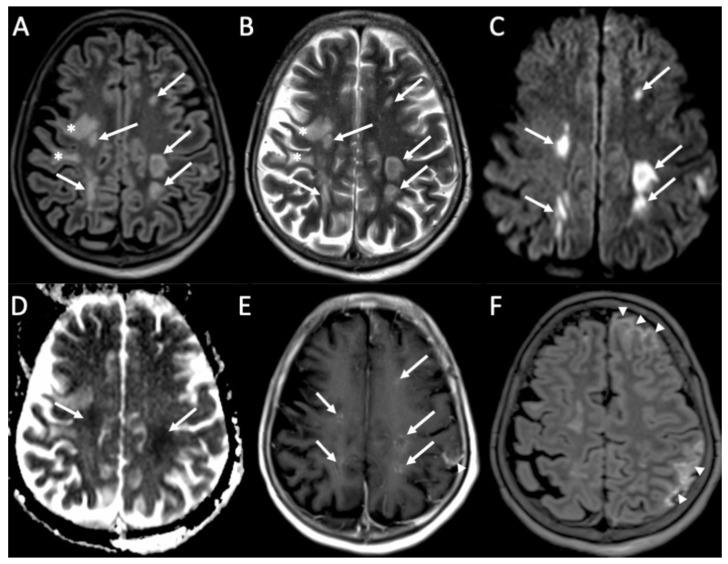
Axial FLAIR (A), T2 (B), DWI (C), ADC (D), enhanced T1 (E), and FLAIR (F) of a patient with Streptococcus Pneumoniae depict multiple recent ischemic areas in the white matter in the fronto-parietal regions bilaterally (arrows in A–E), which are hyperintense on FLAIR and T2, show restricted diffusion and faint contrast enhancement. There is also a leptomeningeal enhancement in the right frontal and parietal regions (arrowheads in E,F). Note also a gliotic area in the right frontal region (*) due to a known previous ischemic event.
